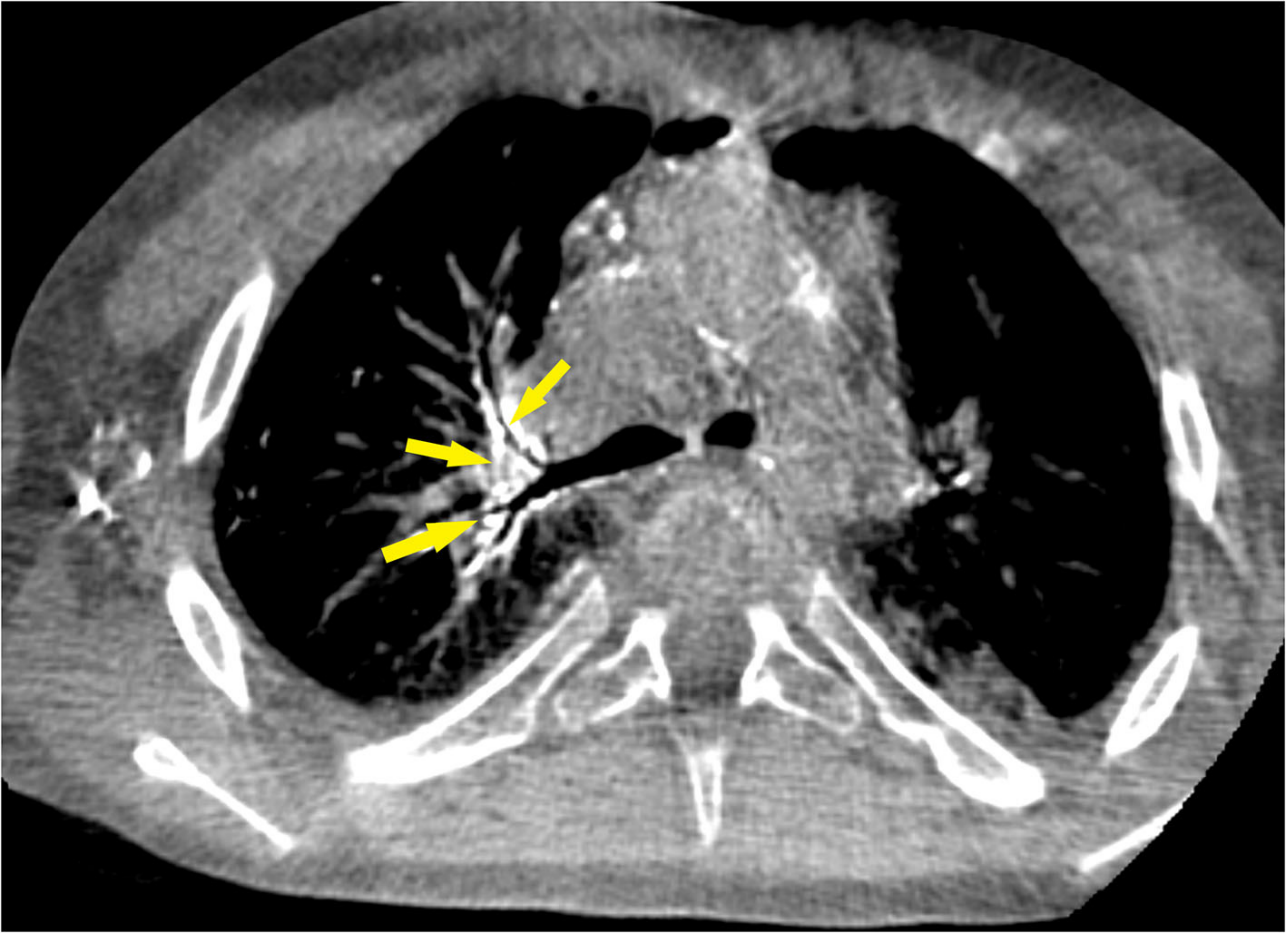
Cone-beam computed tomography image during Lipiodol-based lymphangiography shows filling of several mediastinal abnormal dilated lymph vessels, predominantly peribronchial right (yellow arrows). This is similar to the fluoroscopic image of Figure 1.
